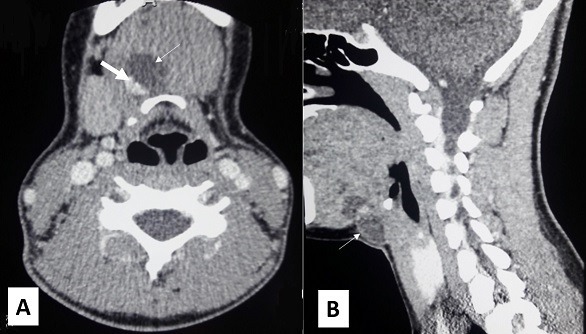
Axial (A) and sagittal (B) with contrast-enhanced computed tomography scan showed a well-circurcumbed cystic lesion attached to the hyoid bone (thin arrow) with a calcified component (thin arrow)
